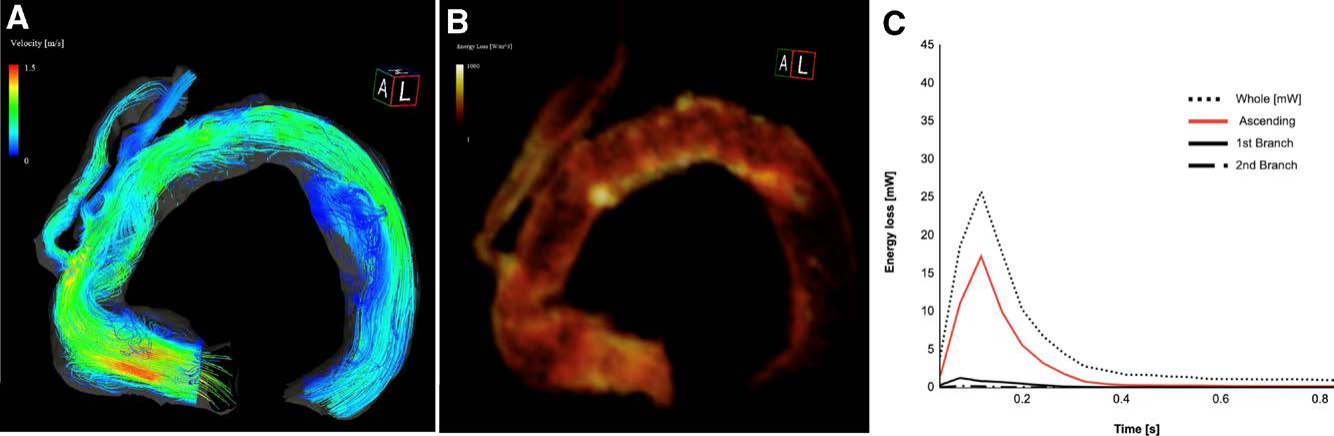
Four-dimensional flow MRI analysis of the streamlines and VEL after the revision surgery. (A, B) The acceleration flow and the bright area disappeared in the acceding portion of the graft. (C) The graph shows that the whole VEL and the VEL at the ascending portion decreased. MRI = magnetic resonance imaging, VEL = viscous energy loss.
